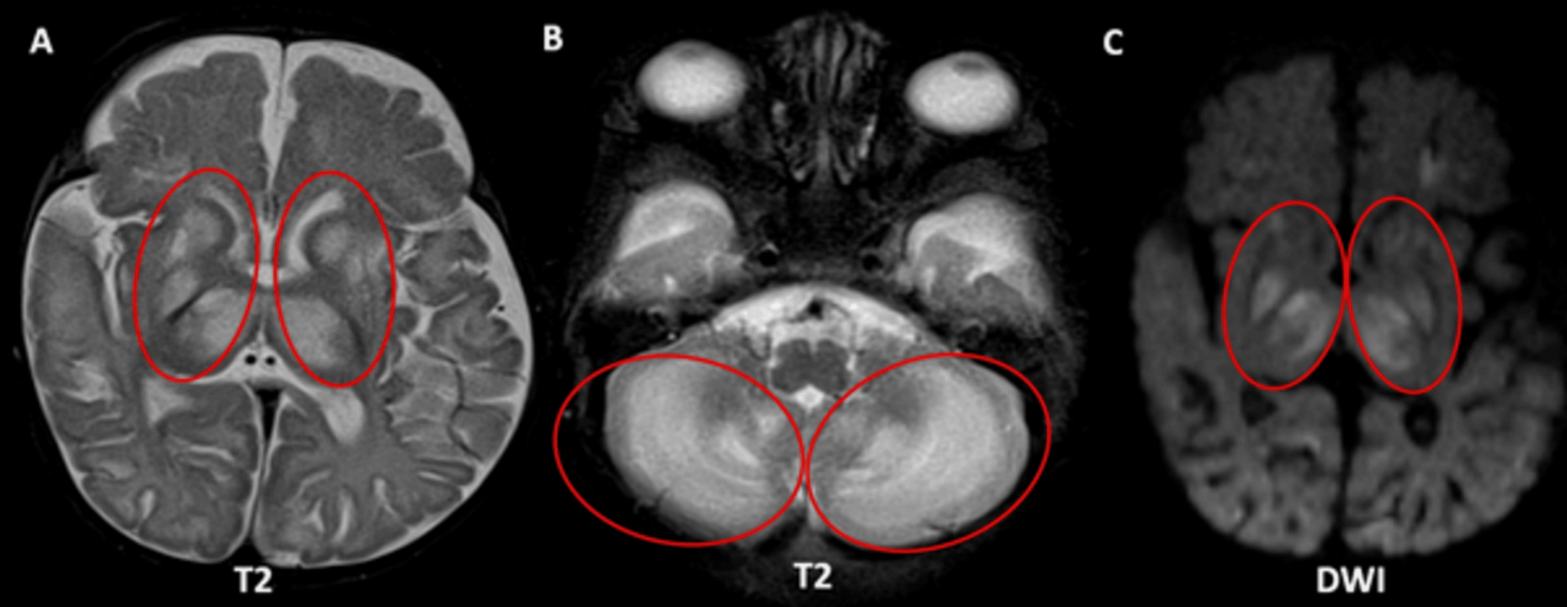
Axial sections of MRI brain (corpus striatum, medial thalami, cerebellar white matter). (A) Multiple areas of T2 hyperintensities in the bilateral corpus striatum and medial thalami (red circles). (B) Multiple areas of T2 hyperintensities in the cerebellar white matter (red circles). (C) Corresponding areas of abnormal restricted diffusion on DWI (red circles). MRI: magnetic resonance imaging; DWI: diffusion-weighted imaging
